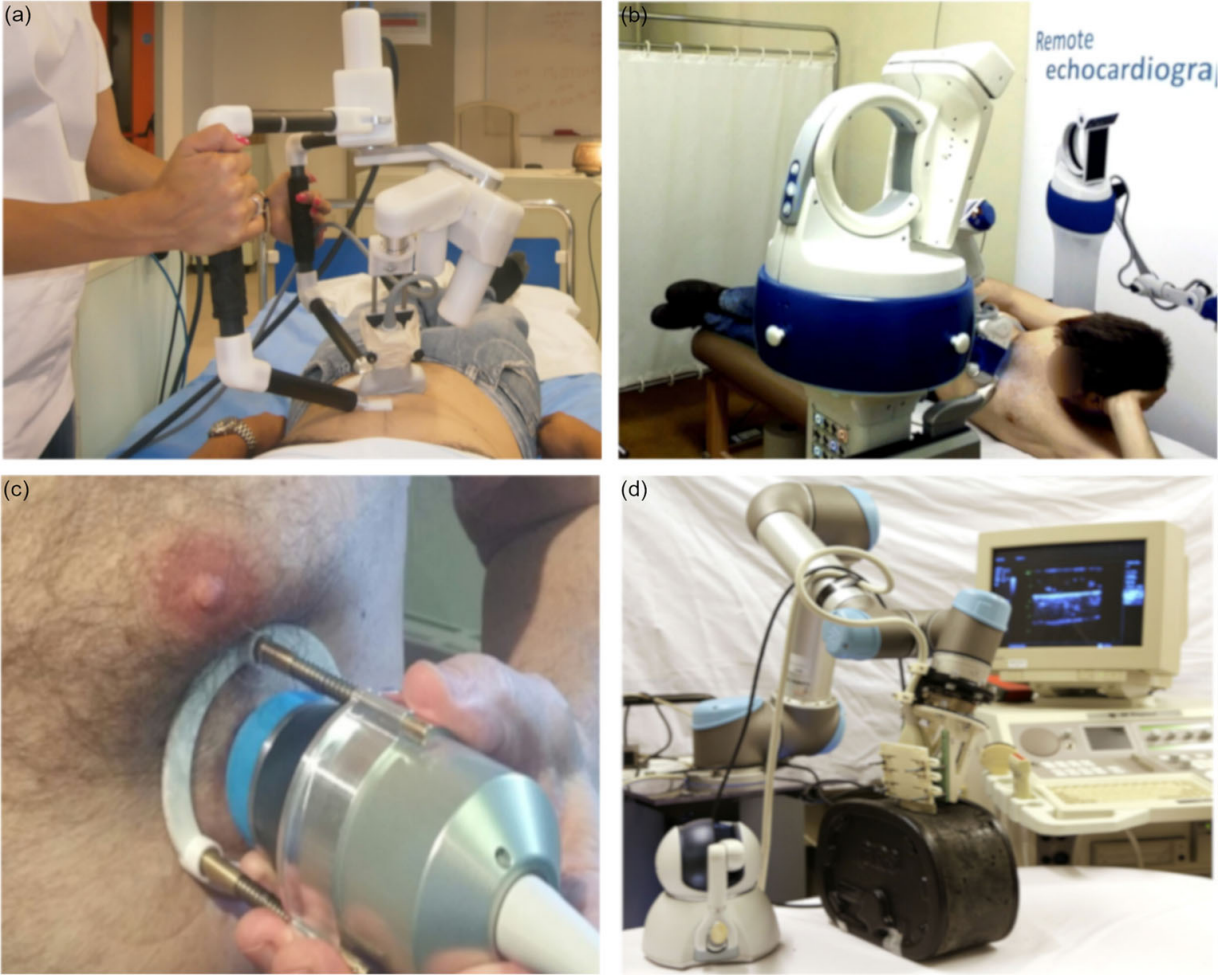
Overview of different teleoperated robotic ultrasound systems. a
